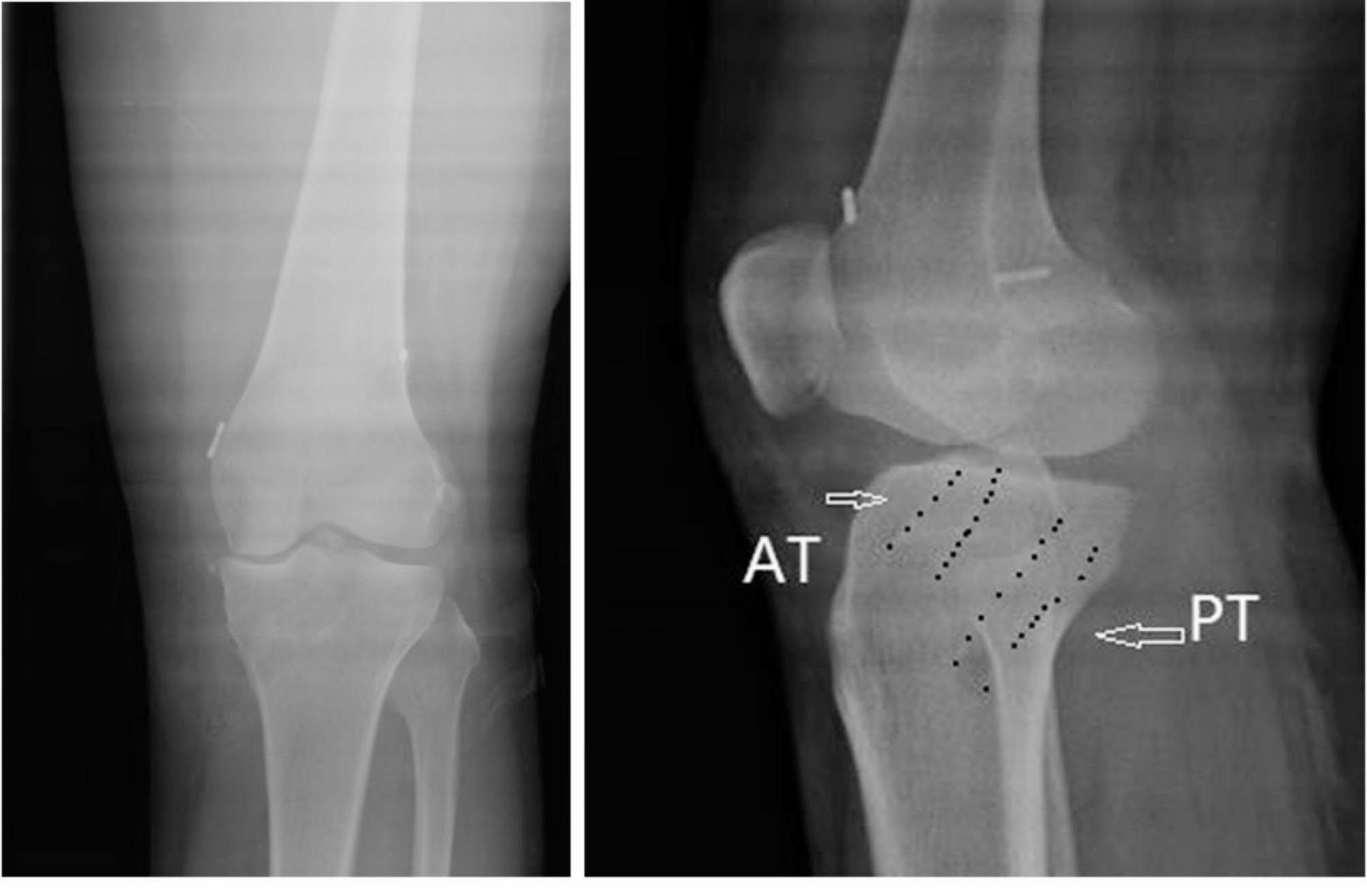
X-ray images revealed that the internal orifice of anterior cruciate ligament tunnel (AT) and posterior cruciate ligament tunnel (PT) on the lateral radiograph is similar to the tibial attachment point of the normal anterior and posterior cruciate ligament after the surgery
